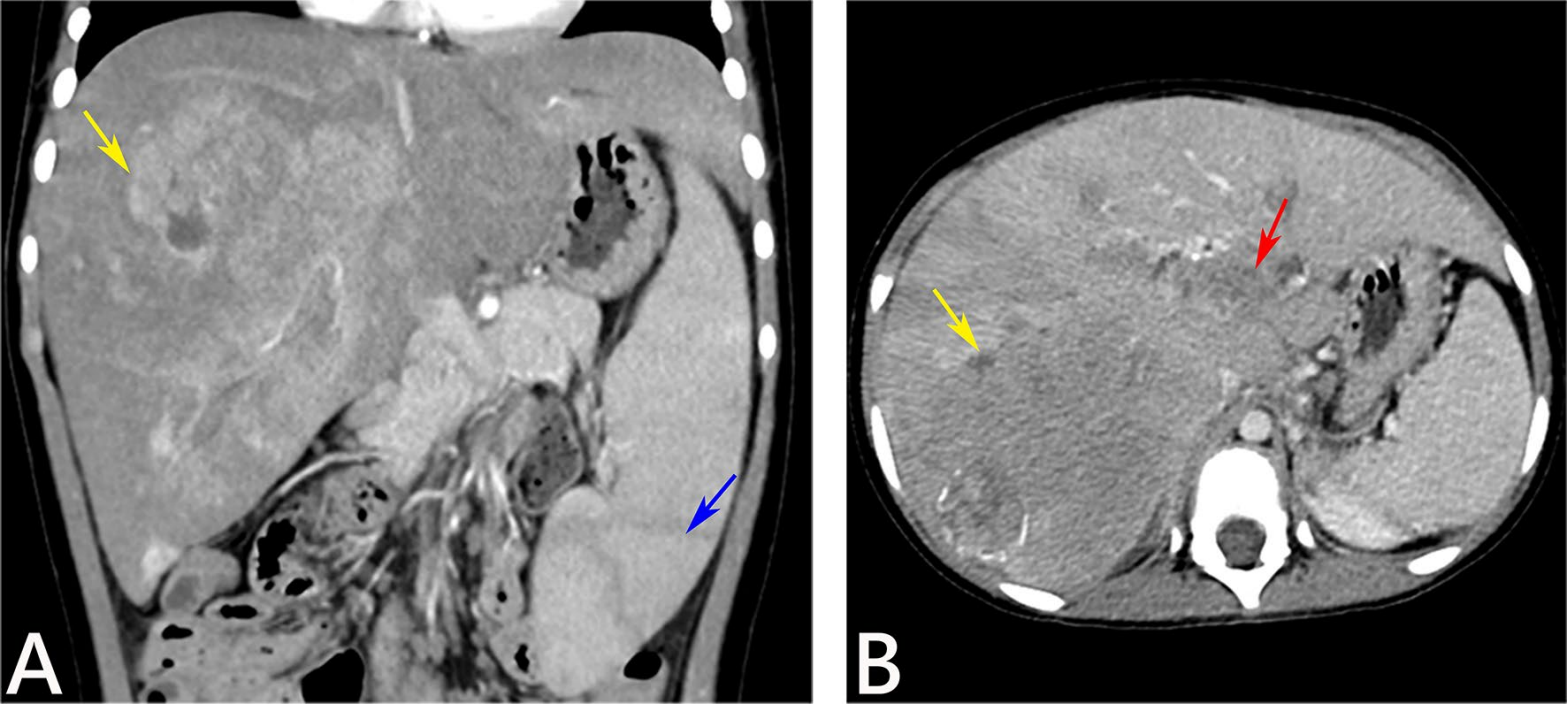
Abdominal enhanced CT findings of the patient at the age 4 years and 9 months. Coronal and axial contrast-enhanced CT images in arterial phase (A) demonstrated a large mass in the right lobe of the liver with the heterogeneous arterial enhancement (yellow arrow) and the enlarged spleen (blue arrow), while portal-venous phase (B) showed a large mass in the right lobe of the liver with wash out (yellow arrow) and a tumor thrombus within the portal vein (red arrow)
